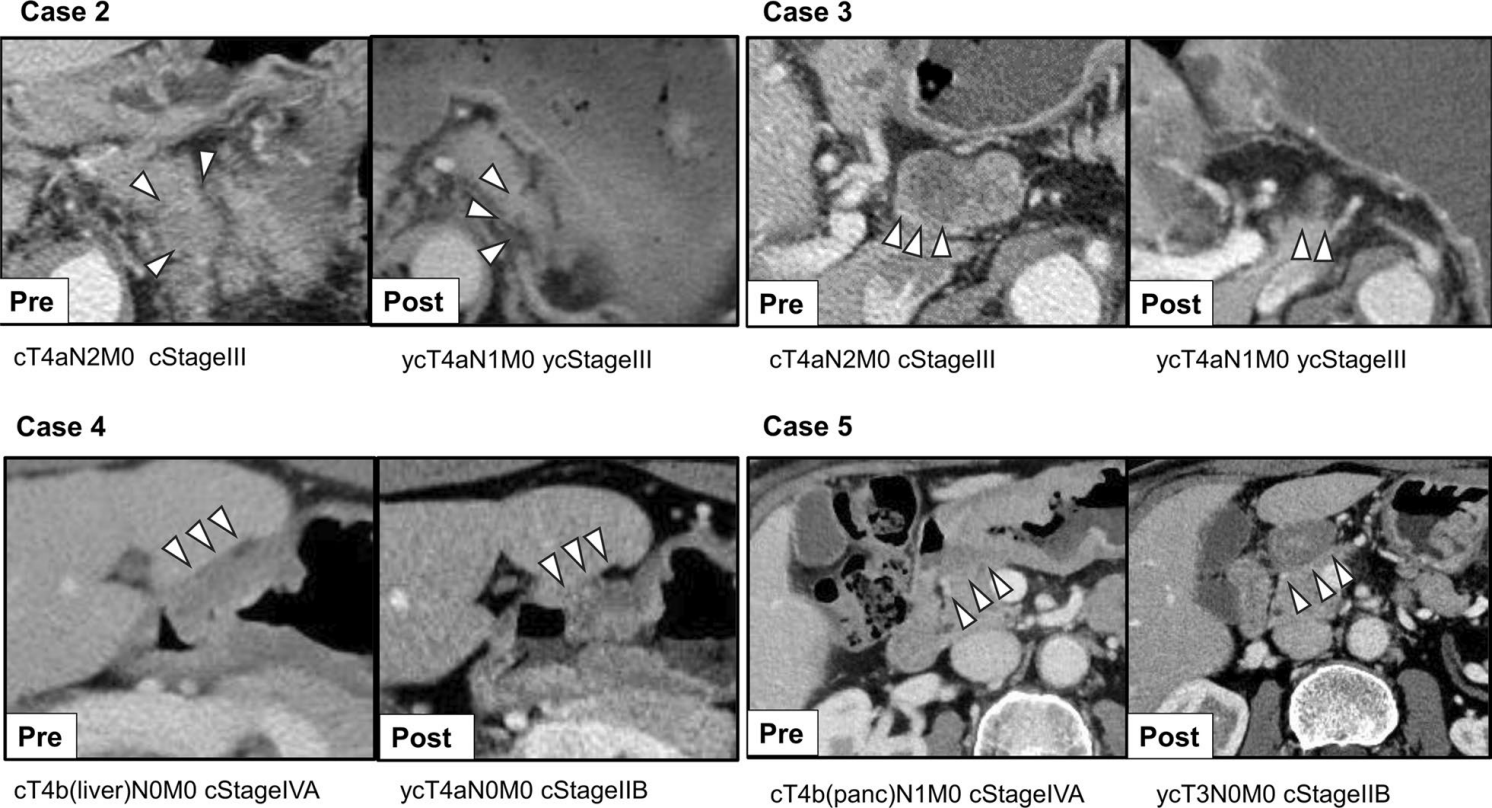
Comparison of computed tomography (CT) scans before and after perioperative chemotherapy. We administered perioperative chemotherapy with nivolumab for locally advanced gastric cancer. The indications for perioperative chemotherapy were as follows: lymph node adjacency to the pancreas (Case 2), lymph node adjacency to the liver (Case 3), tumor adjacency to the liver (Case 4), and tumor adjacency to the pancreas (Case 5). An objective response with major tumor shrinkage was observed in all patients
